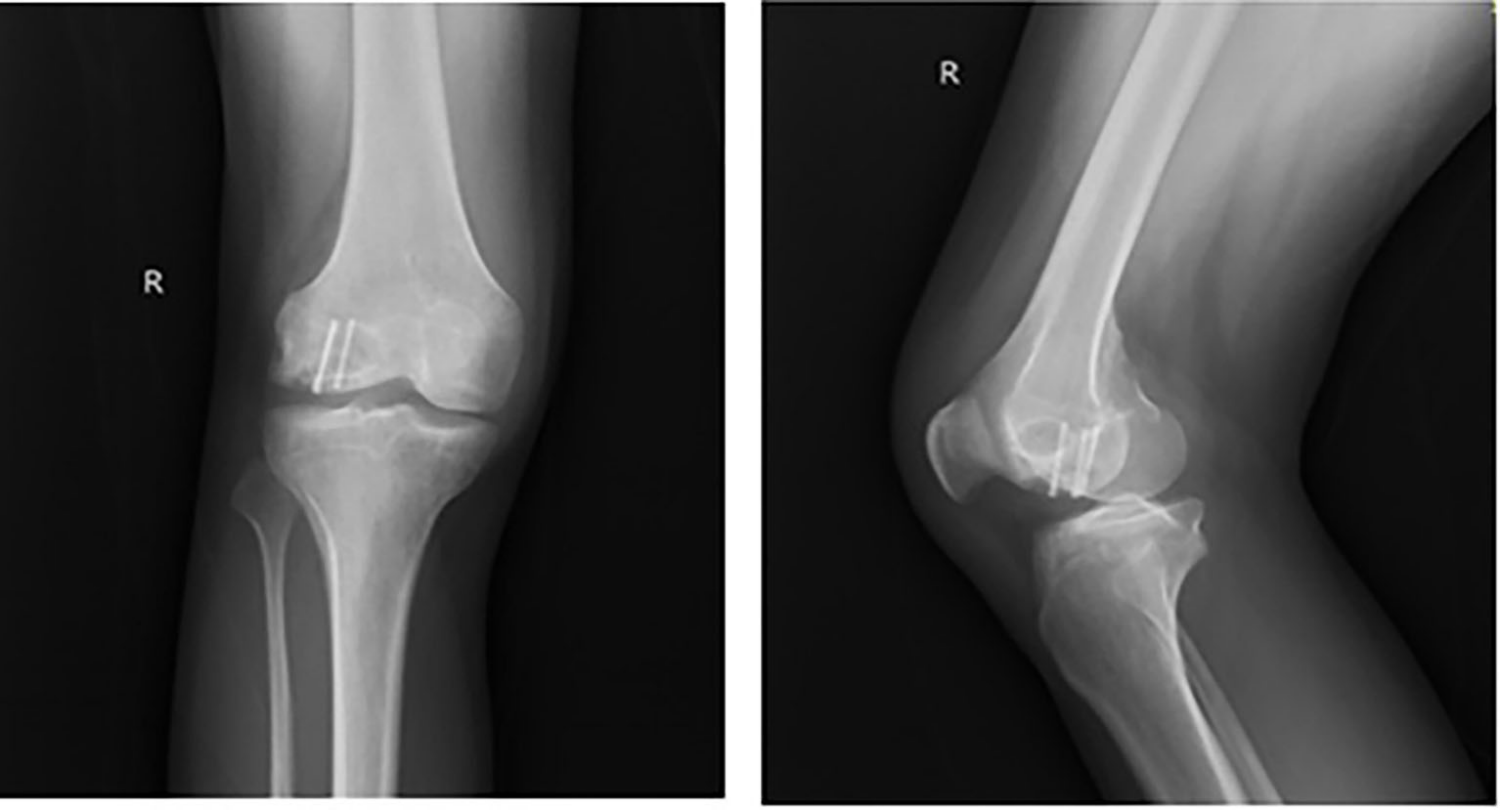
Pinning procedure seen on the X-ray imaging of the patient.
